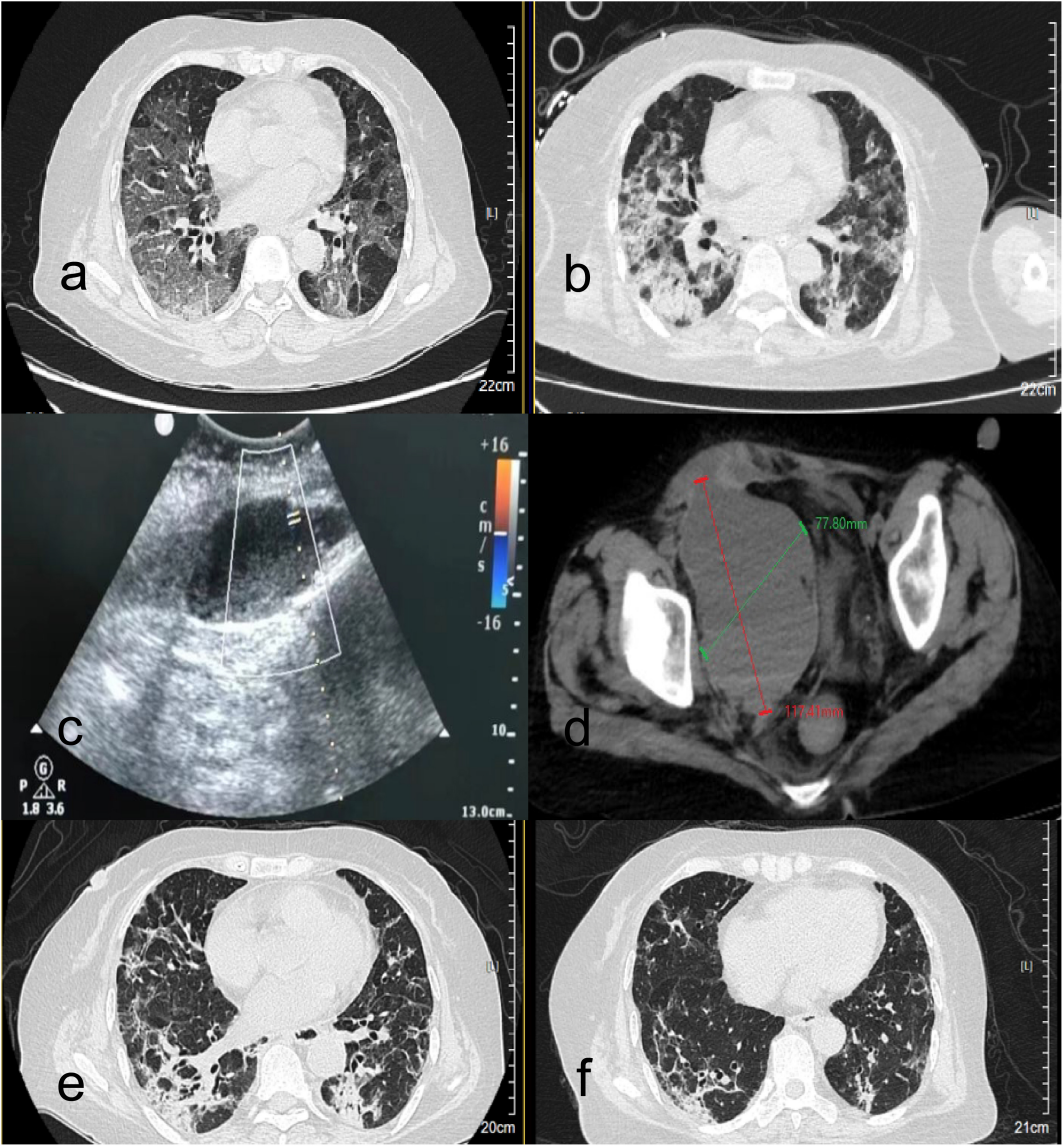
Radiological findings in a COVID-19 patient with membranous nephropathy during hospitalization. (a,b) Serial axial chest CT images (lung window). (a) Non-contrast CT on admission (day 1) reveals extensive bilateral multifocal ground-glass opacities and consolidations, predominantly distributed in peripheral and subpleural regions. (b) Follow-up CT on day 22 after treatment shows marked resolution of pulmonary opacities. (c,d) Imaging of rectus sheath hematoma. (c) Bedside ultrasonography demonstrates a cystic-solid mass extending from the rectus abdominis into the pelvis, with a maximum depth of 113 mm and poor sound transmission. Color Doppler imaging shows blood flow signals along the posterior wall of the rectus abdominis anterior to the cystic mass; pulse-wave Doppler reveals an arterial spectrum with a peak velocity of 30 cm/s. (d) Abdominal CT depicts a heterogeneous-density hematoma adjacent to the right rectus abdominis, extending into the pelvic cavity, measuring 117.41 mm in longitudinal and 77.80 mm in transverse diameters. (e,f) Follow-up axial chest CT (lung window) on day 35 (e) and day 46 (f), demonstrating continued resolution of pulmonary opacities, with minimal residual subpleural ground-glass infiltrates and near-complete absorption of consolidations.
